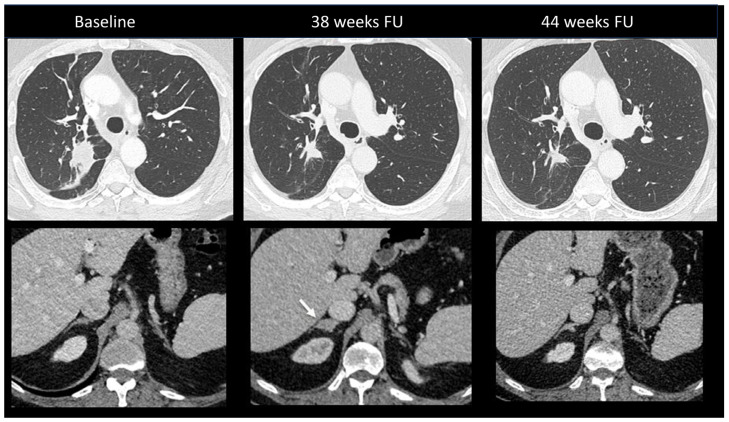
Immune-related adrenalitis in a patient with a lung carcinoma treated with Nivolumab. CT images taken 38 weeks after the start of treatment demonstrate a significant decrease in the size of the primary lung tumor compared to baseline, but an increase in size with a nodular appearance of the right adrenal gland suspected for a new metastasis (arrow). Despite this finding, immunotherapy was continued. Subsequent CT images performed 44 weeks after treatment revealed the disappearance of the enlargement of the right adrenal gland, confirming the diagnosis of immune-related adrenalitis.
